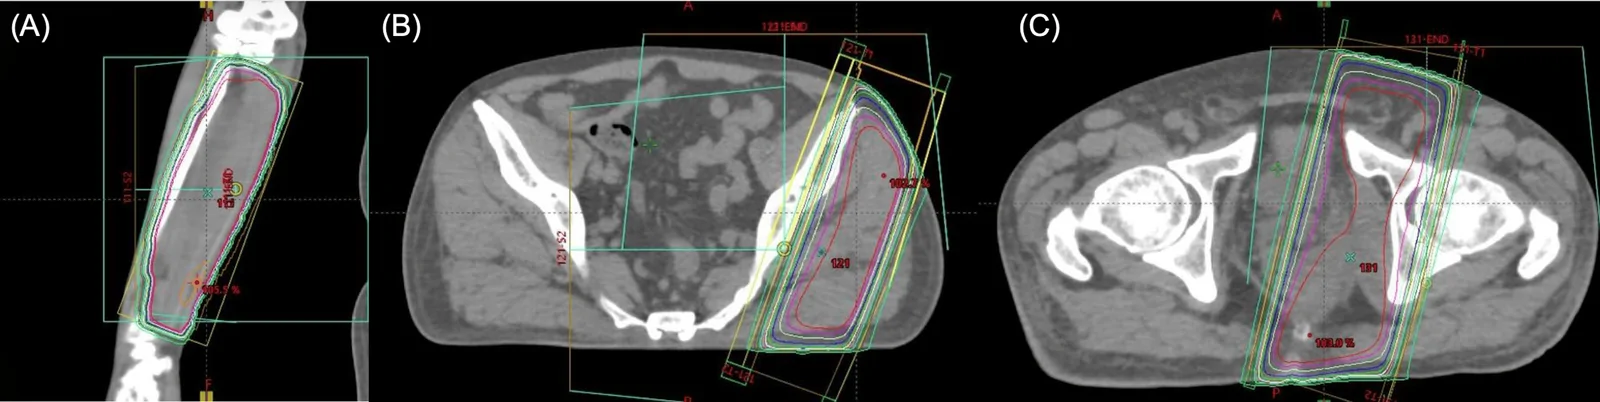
Radiotherapy Treatment Planning CT for the Three Presumed Metastatic Sites Radiotherapy planning CT with dose distributions for the three lesions treated as presumed skeletal muscle metastases: (A) right forearm, (B) left gluteus medius, and (C) left obturator internus. A total dose of 30 Gy in 10 fractions was planned for each site. Treatment to the right forearm was discontinued after 21 Gy in seven fractions when compartment syndrome developed; treatment to the left gluteus medius and left obturator internus was discontinued after 27 Gy in nine fractions when nocardiosis was suspected.
